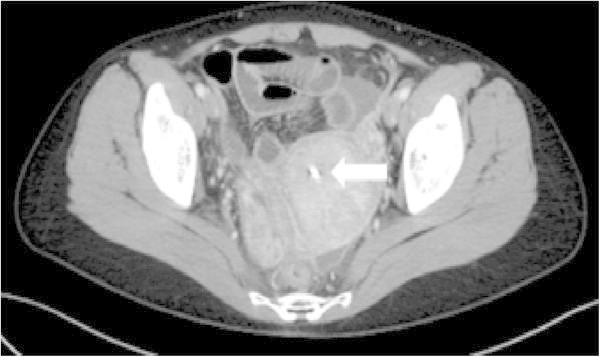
Plain CT of the pelvic region. White arrow: Intrauterine foreign body.
